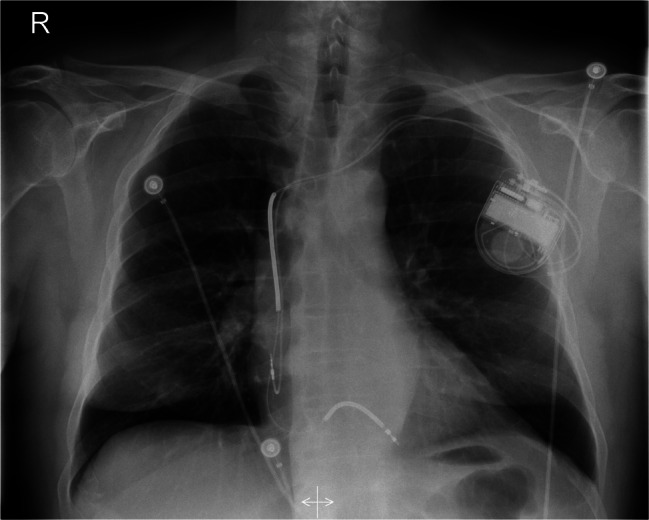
X-ray: placement of a DDD-ICD-system in the same session, with a new ventricular lead in the right apex and a new atrial lead in the right atrial appendage
